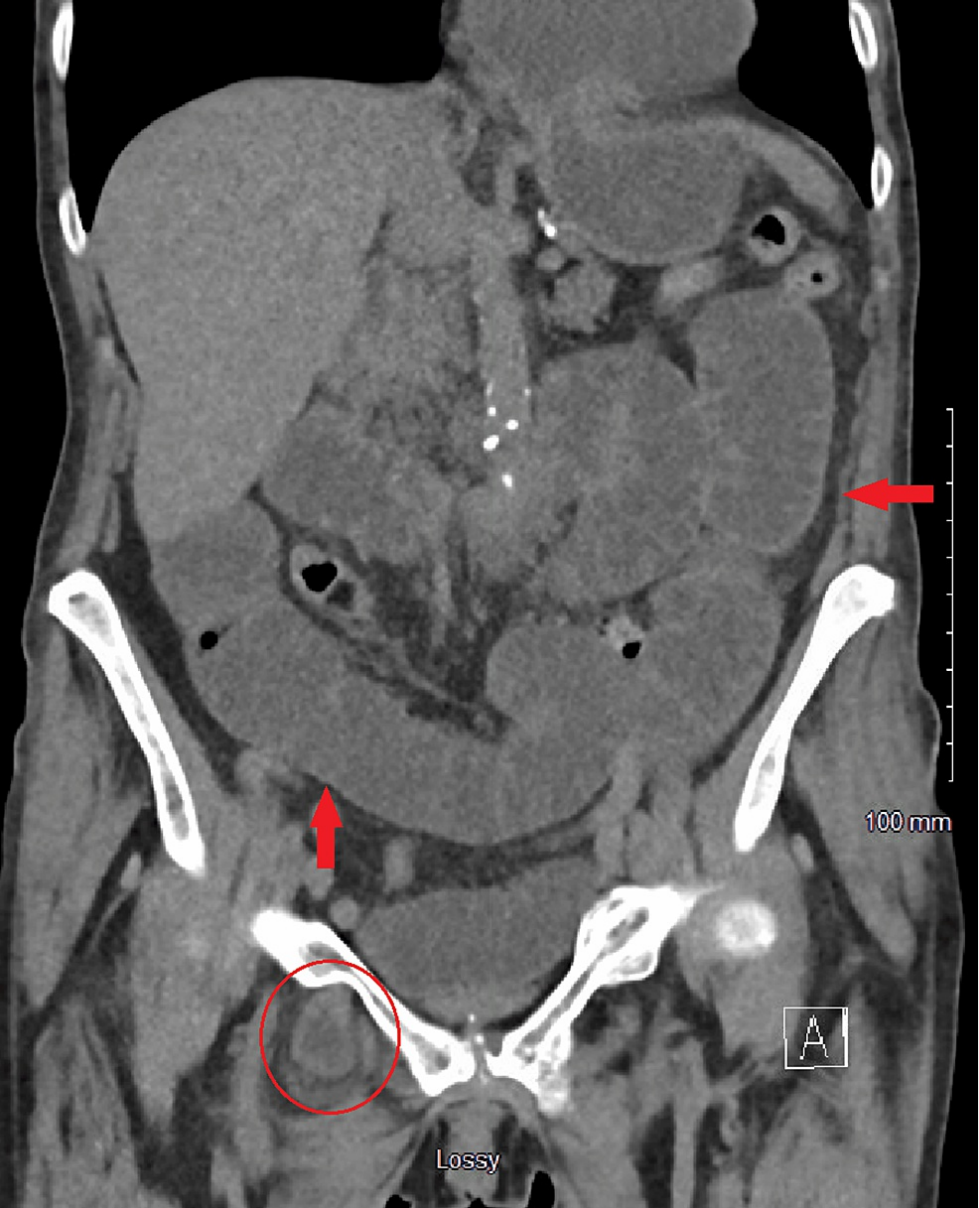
Coronal computed tomography on initial presentation in 2018 with incarcerated right obturator hernia (red circle) and dilated small bowel loops (red arrows), suggestive of small bowel obstruction.
